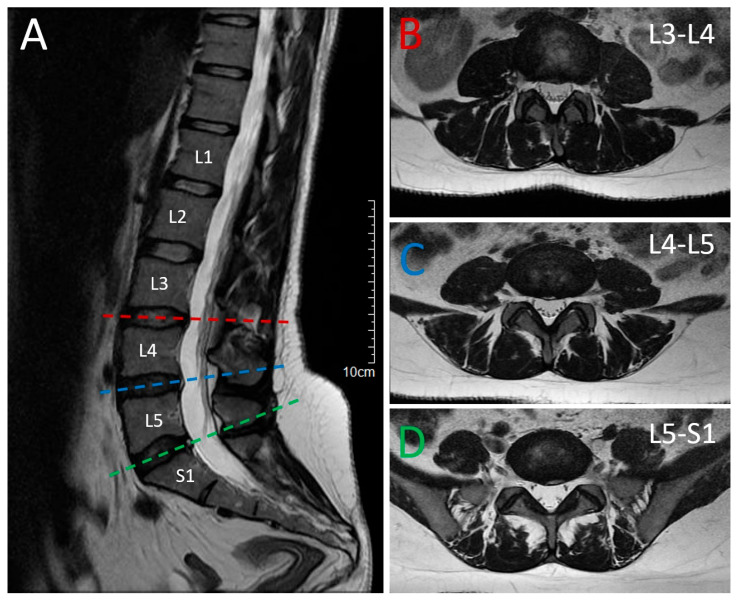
Magnetic Resonance Imaging (MRI) of the lumbar spine. (A) T2-weighted sagittal spine magnetic resonance imaging (MRI)showing degenerative changes in the lumbar spine with multilevel bulging of the intervertebral discs, osteochondrosis. Horizontal lines indicate the corresponding levels in the axial views. (B–D) T2-weighted axial spine MRI at different levels presenting the degenerative changes and intervertebral disc protrusions L3–L4, L4–L5 and L5–S1.
